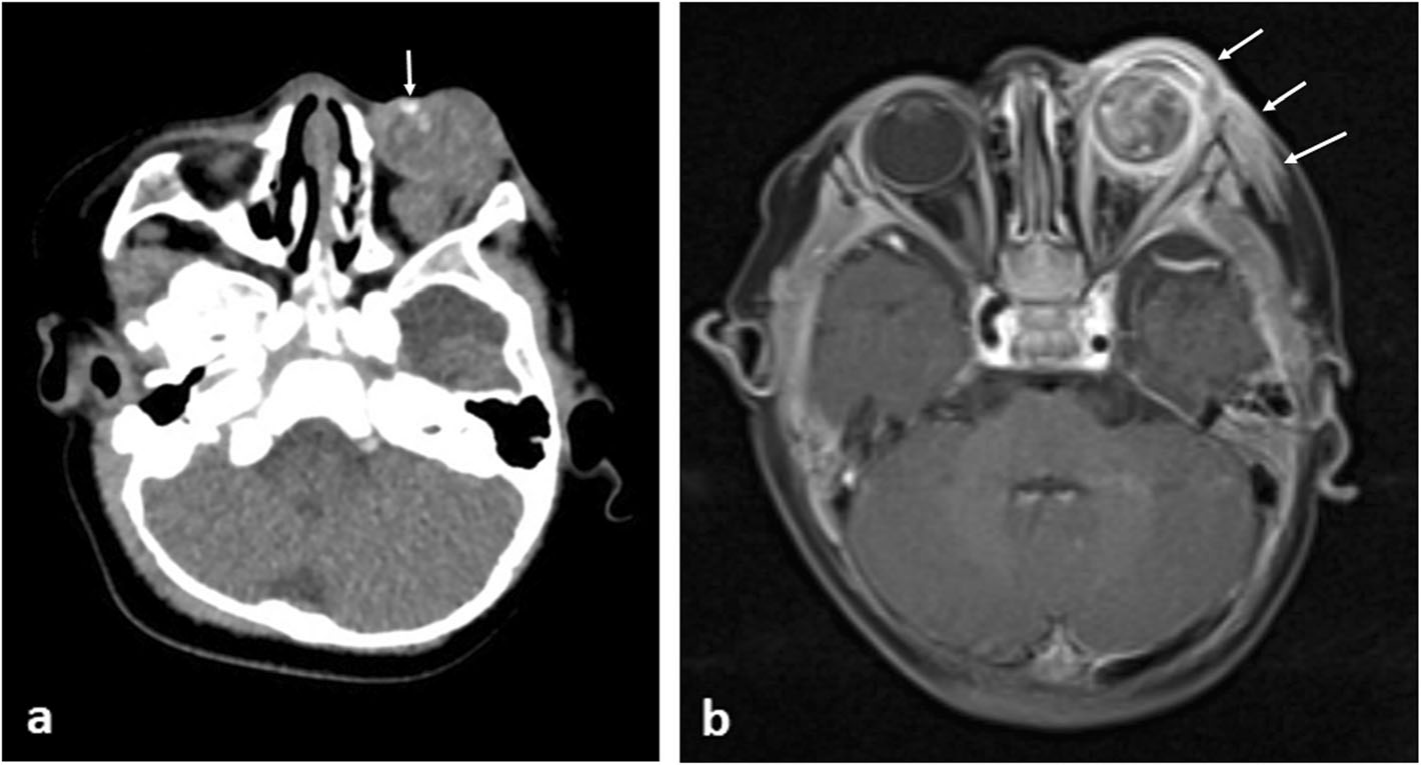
A 6-month-old male with biopsy proven intraocular rhabdoid tumour. a Axial CT showing a large left intraorbital mass involving mainly the intraconal compartment with associated marked proptosis. The lesion demonstrates internal calcifications (arrow). b Post-contrast fat-saturated T1-weighted MR image shows intra and periorbital abnormal contrast enhancement (arrows)
